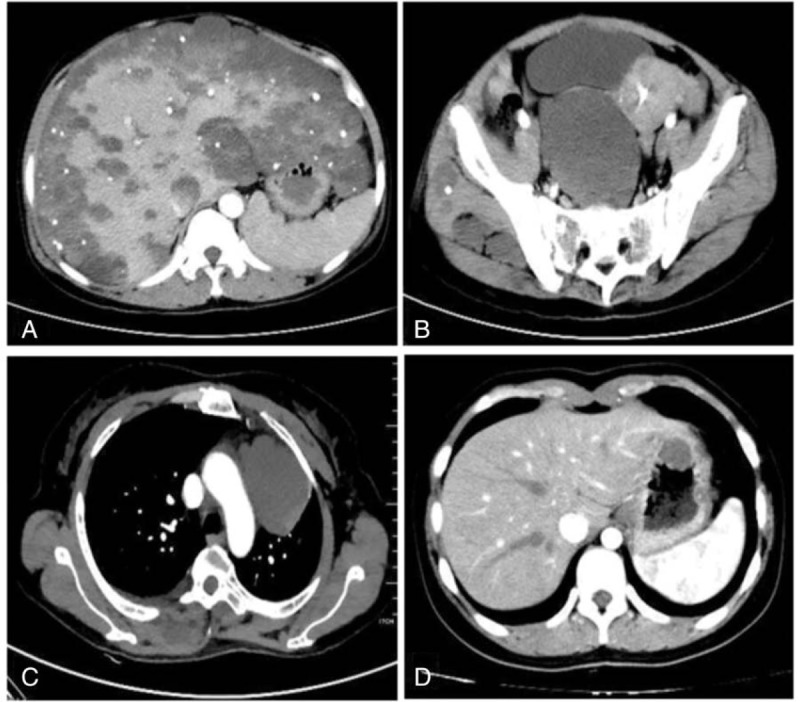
Abdomen CT scan with intravenous contrast administration revealed multiple vascular malformations on the liver (A), muscles of buttocks and pelvic cavity (B), mediastinum (C), and stomach (D), and multiple punctate calcification could be seen in the lesions. CT = computed tomography.
